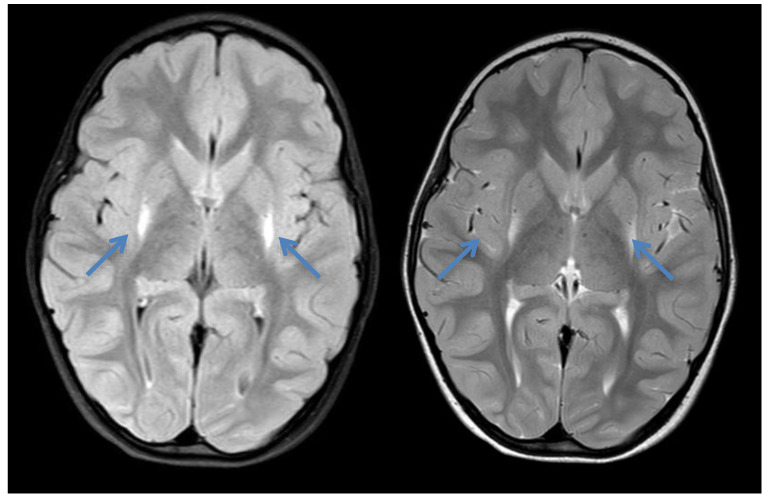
Cranial MRI of patient 3 at the age of 4 years showing increased signal intensity bilaterally around the putamen (blue arrows) in axial T1 and T2—the typical insidious onset pattern of GA-1.
